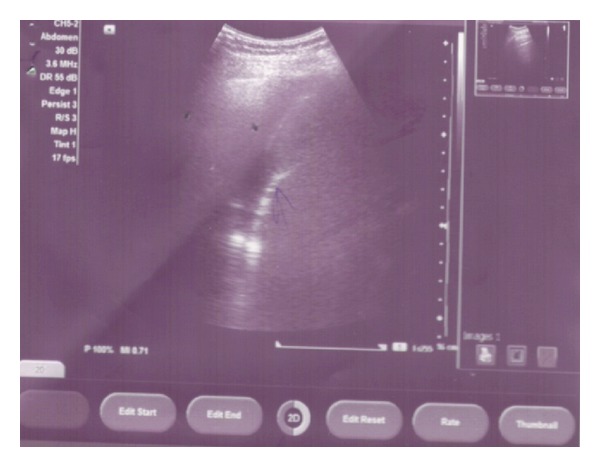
Thoracic ultrasound shows effusion in the right pleural space.
